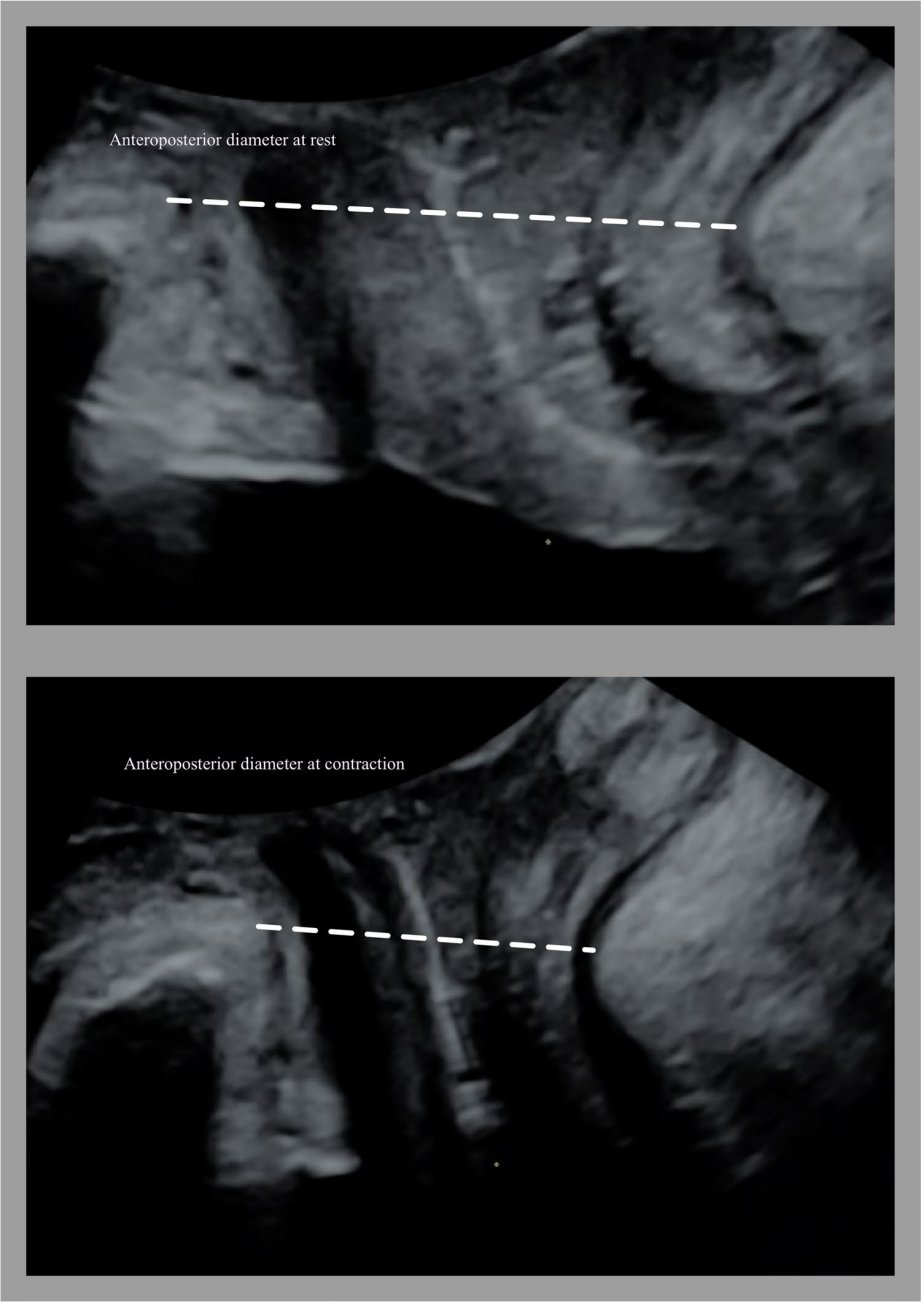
Assessment of pelvic floor contraction by ultrasound. Measurement of the anteroposterior diameter of the levator hiatus as the distance from the symphysis pubis to the puborectalis muscle at rest (*top*) and contraction (*bottom*)
